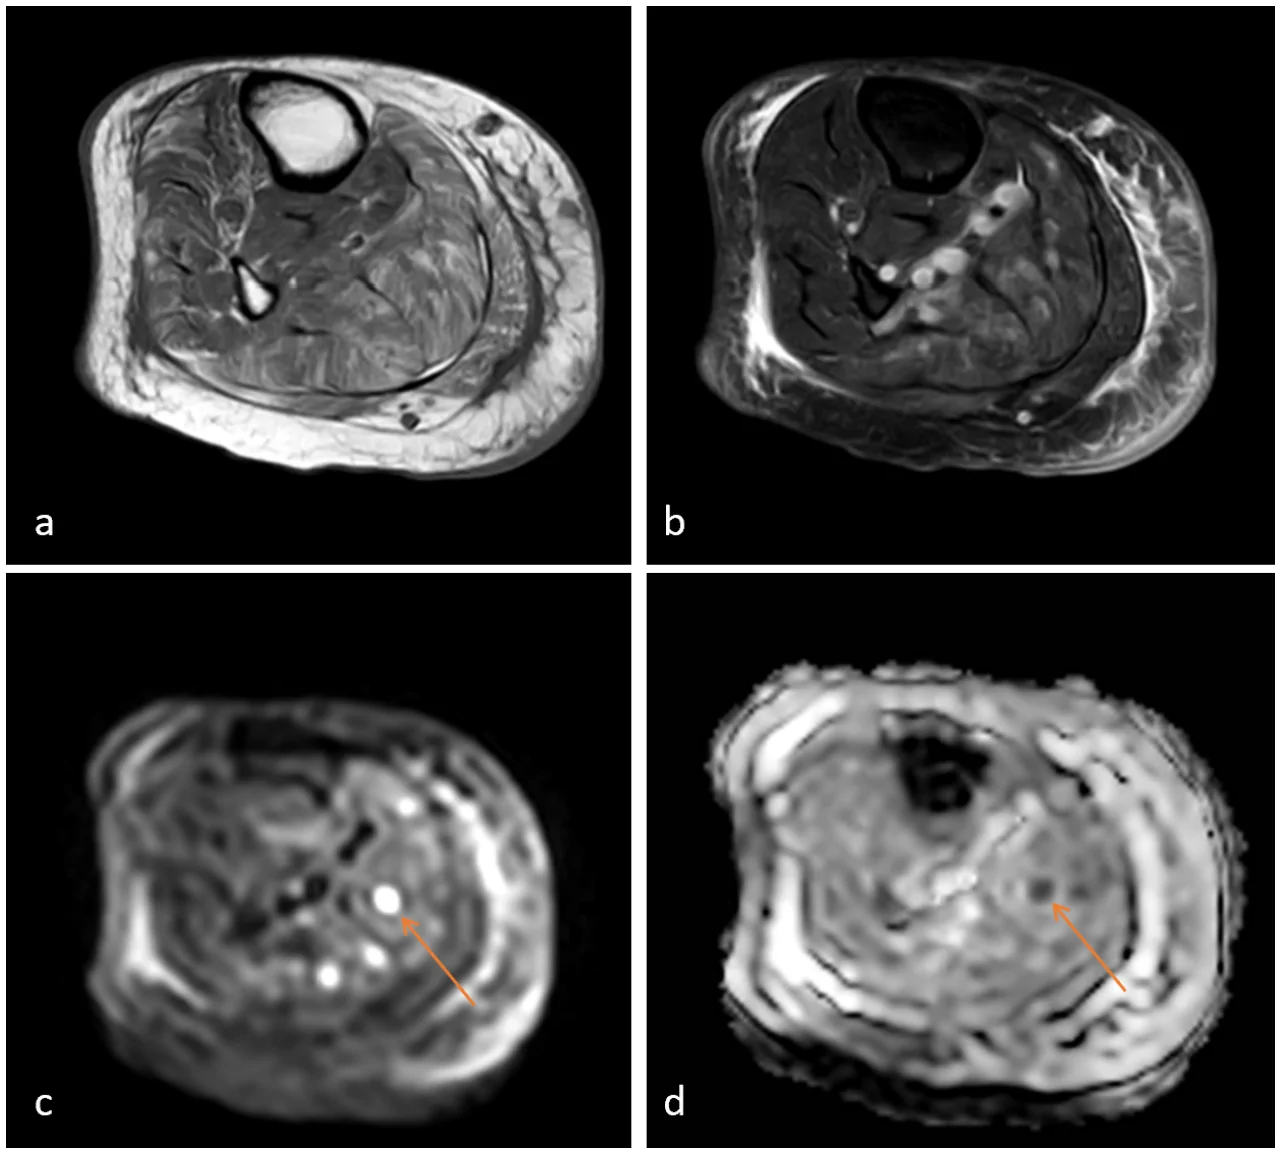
Axial MRI of a patient with cellulitis demonstrating an incidentally detected acute soleal vein thrombosis (image-based diagnosis only). The acute thrombus within the soleal vein is inconspicuous on T1- and T2-weighted sequences (a,b) but is clearly identified as a focus of high signal on DWI with corresponding restricted diffusion on the ADC map (c,d) (orange arrows), highlighting the additive value of DWI in the detection of acute deep vein thrombosis.
